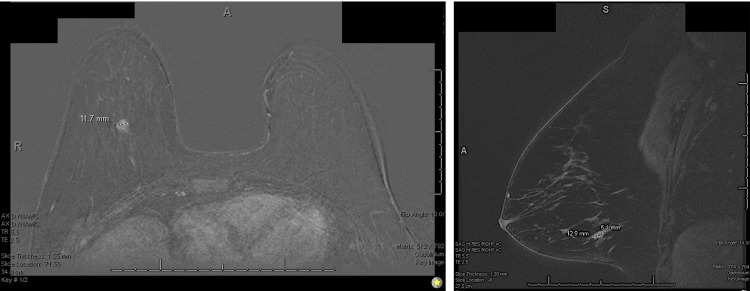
Bilateral breast MRI demonstrating tumor dimensions. T2-weighted images demonstrate a T2 hyperintense biopsy marker abutting the inferior margin of a mildly T2 hyperintense 12 x 13 x 5 mm rapidly enhancing mass at 6 o'clock indicated in the dotted line, middle to posterior depth of the right breast. No other rapidly enhancing or suspicious abnormalities were identified. No lymphadenopathy was identified in the right axilla.
